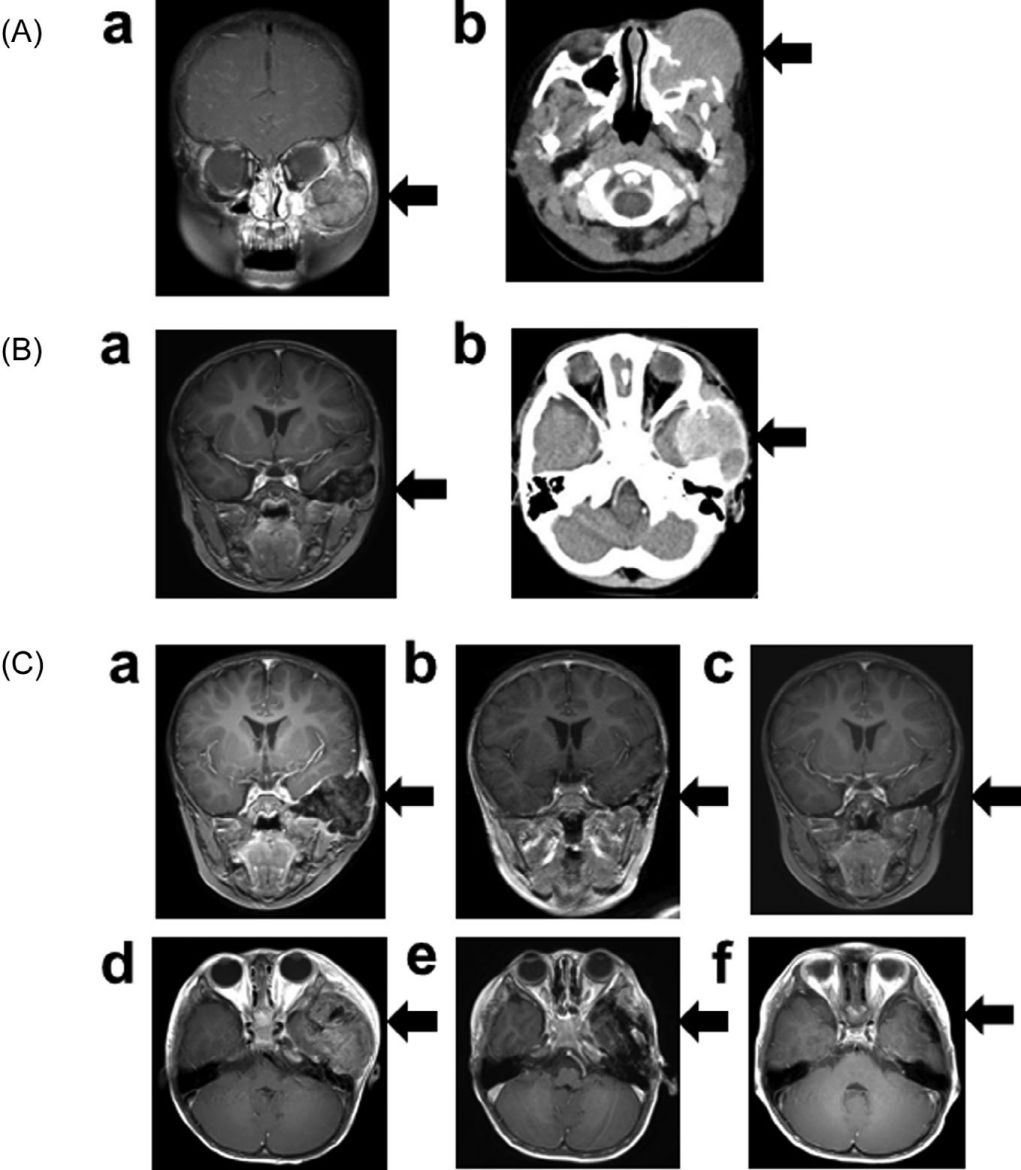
Clinical presentation of patient 1 showing primary and recurrent sites of LCH that were resolved by first CLO monotherapy. (A) The primarily involved sites of the left lower orbital wall and the maxillary sinus are shown by (a) gadolinium (Gd)‐enhanced magnetic resonance imaging (MRI) and (b) enhanced computed tomography (CT) axial images. (B) The recurrent lesion of the left sphenoidal bone is shown by (a) Gd‐enhanced MRI and (b) enhanced CT images. (C) Clinical response to CLO monotherapy is shown by Gd‐enhanced MRI (a) and (d) before treatment, (b) and (e) after two cycles of CLO, and (c) and (f) after the completion of 12 cycles of CLO. Arrows indicate each site of involved lesions
